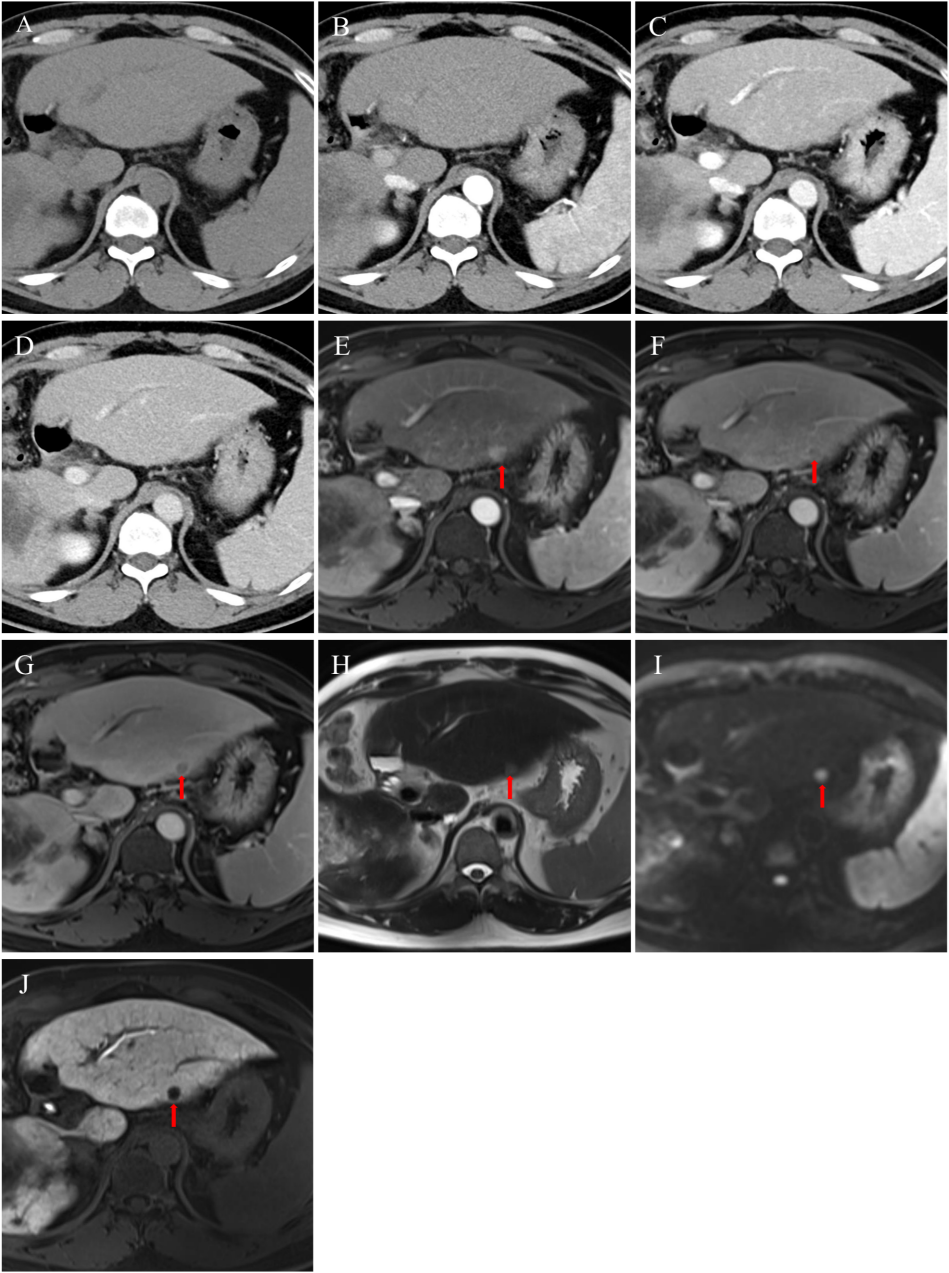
A case of a 50-year-old male patient with a history of hepatitis B-related cirrhosis, presenting with 11-month follow-up imaging after curative microwave ablation (MWA) for hepatocellular carcinoma (HCC). Initial multiphase contrast-enhanced CT (A–D) showed no definite signs of intrahepatic recurrence. However, comparative analysis of the same level using an additionally performed Gd-EOB-DTPA-enhanced MRI (E–J) revealed a approximately 1.3 cm lesion in segment III of the left hepatic lobe. The imaging characteristics of this lesion were consistent with HCC: arterial phase hyperenhancement (E), "washout" appearance (hypointensity) in the portal venous (F) and delayed phases (G), mild hyperintensity on T2-weighted imaging (H), restricted diffusion on diffusion-weighted imaging (I), and hypointensity on the hepatobiliary phase (J). The lesion was ultimately confirmed as an intrahepatic HCC recurrence.
